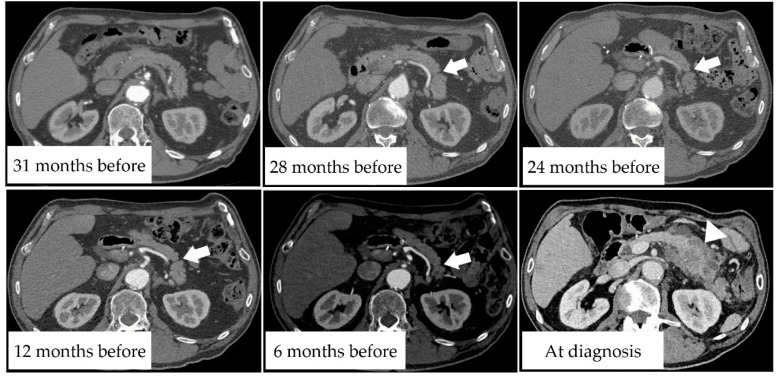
A man in his late 70 s with pancreatic tail ductal adenocarcinoma. Pre-diagnostic CT scans at 31, 28, 24, 12, and 6 months before PDAC diagnosis showing temporal changes in the pancreatic tail. White arrows indicate focal pancreatic atrophy, and the white arrowhead indicates invasive pancreatic ductal adenocarcinoma.
